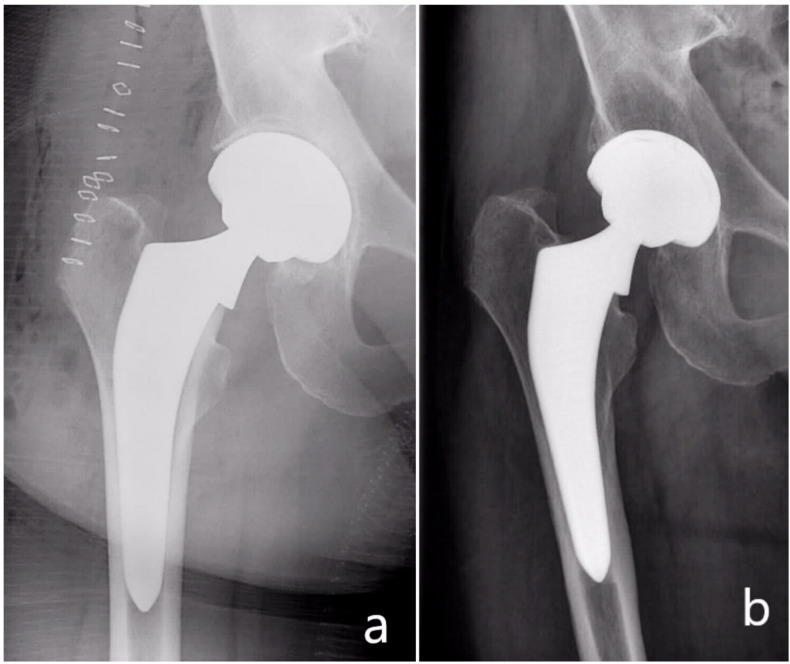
(a) Postoperative radiograph, (b) radiograph of the same patient at three months showing subsidence of the stem.
